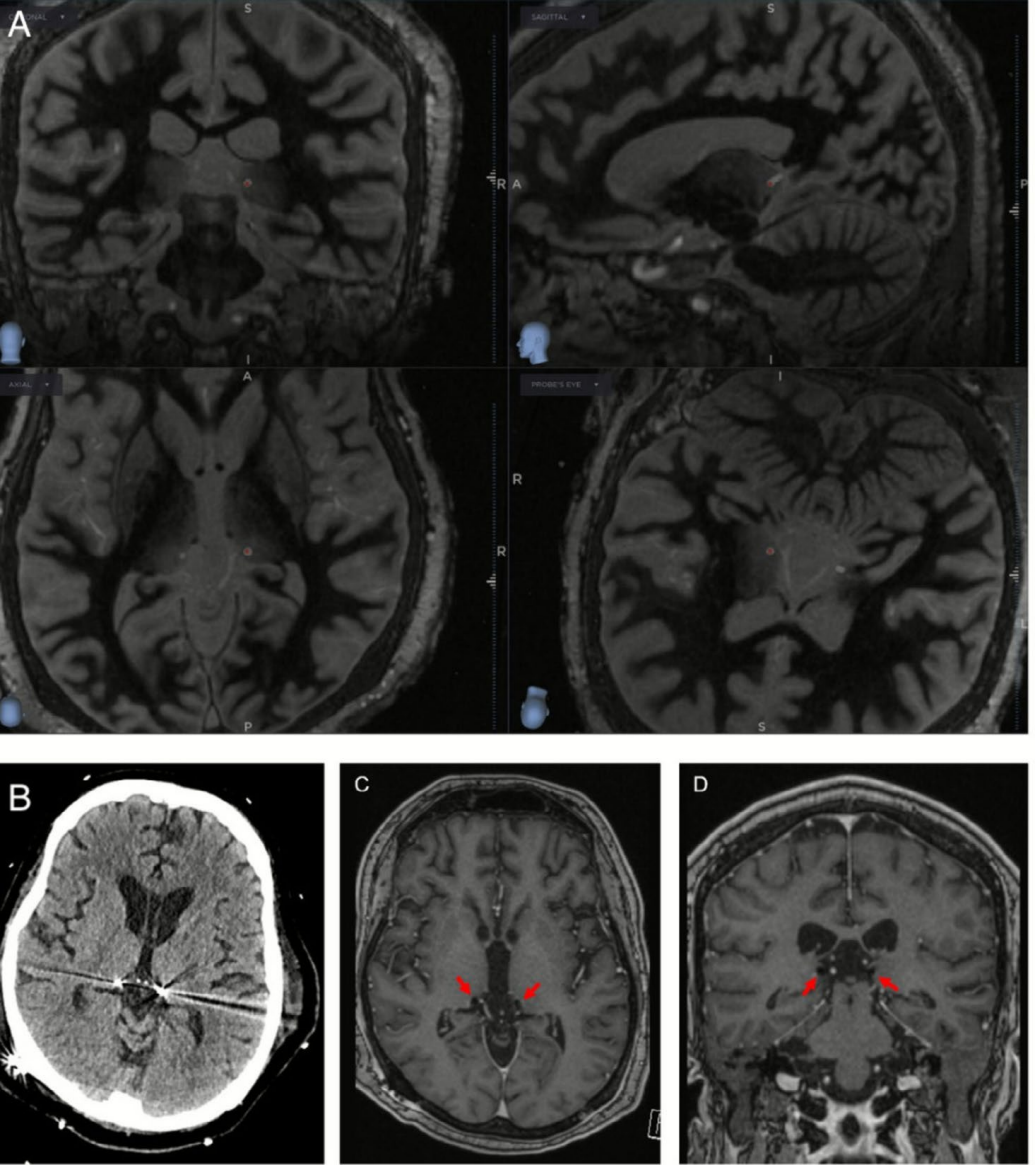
Intraoperative 3Tesla MRI brain with Fast Gray Matter Acquisition T1 Inversion Recovery (FGATIR) sequences documenting DBS lead placement (A). Follow up CT brain on day one (B) and MRI brain 2 months (C and D) post‐op.
